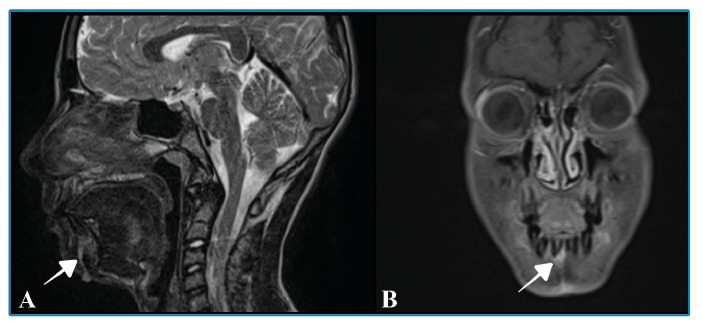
A: Sagittal T2-weighted magnetic resonance (MR) images showing a sinus tract (arrow) crossing the mandible and opening into the skin, a manifestation of osteomyelitis. B: Coronal fat-supressed contrast-enhanced T1-weighted MR images showing enhancement in the mandibular corpus at the alveolar level.
